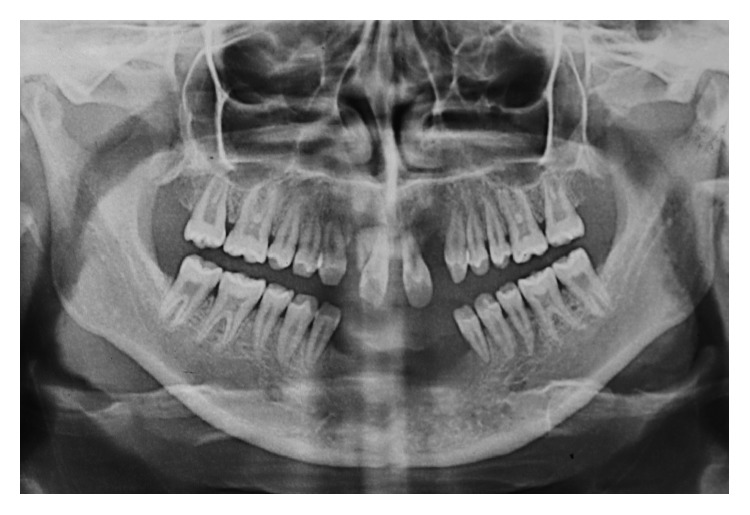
Panoramic radiograph showing agenesis of maxillary lateral incisors, mandibular incisors, and all 4 third molars.
